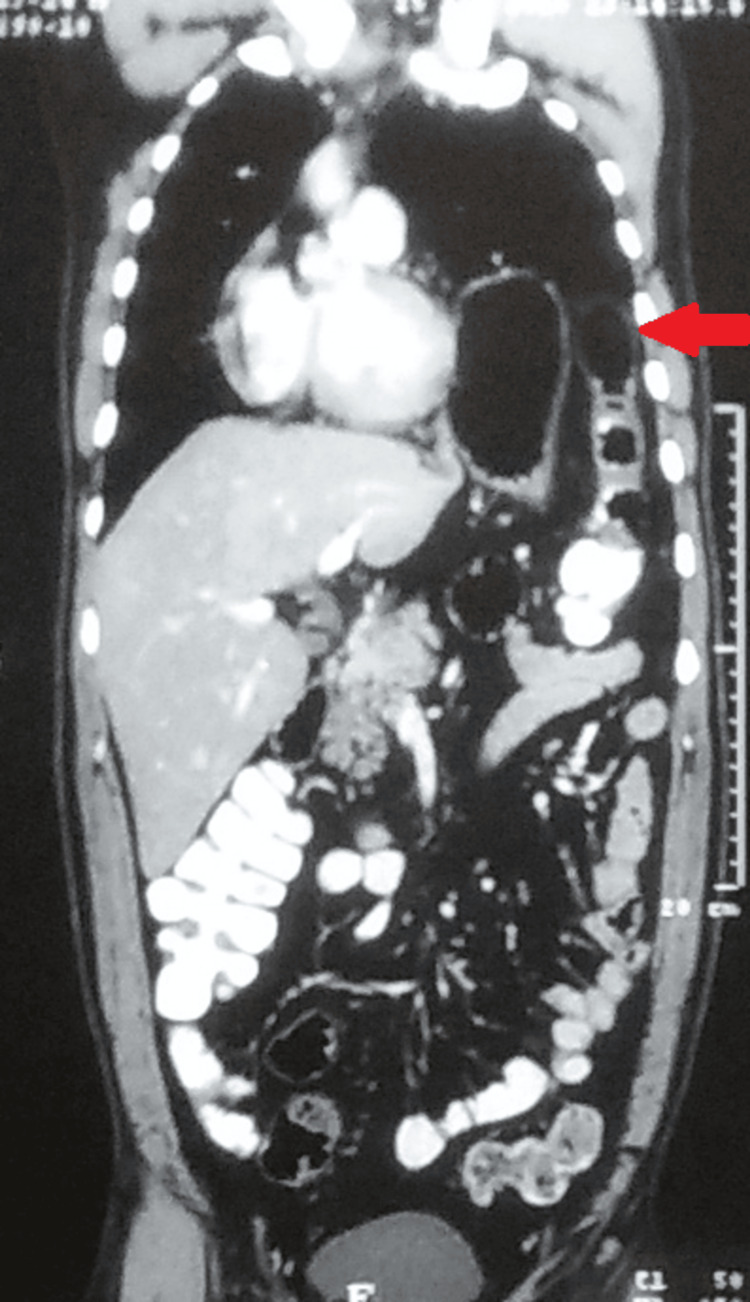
Contrast-enhanced CT of the abdomen. Arrow suggestive of a raised left hemidiaphragm with a superiorly displaced spleen, stomach, and splenic flexure of the colon.
